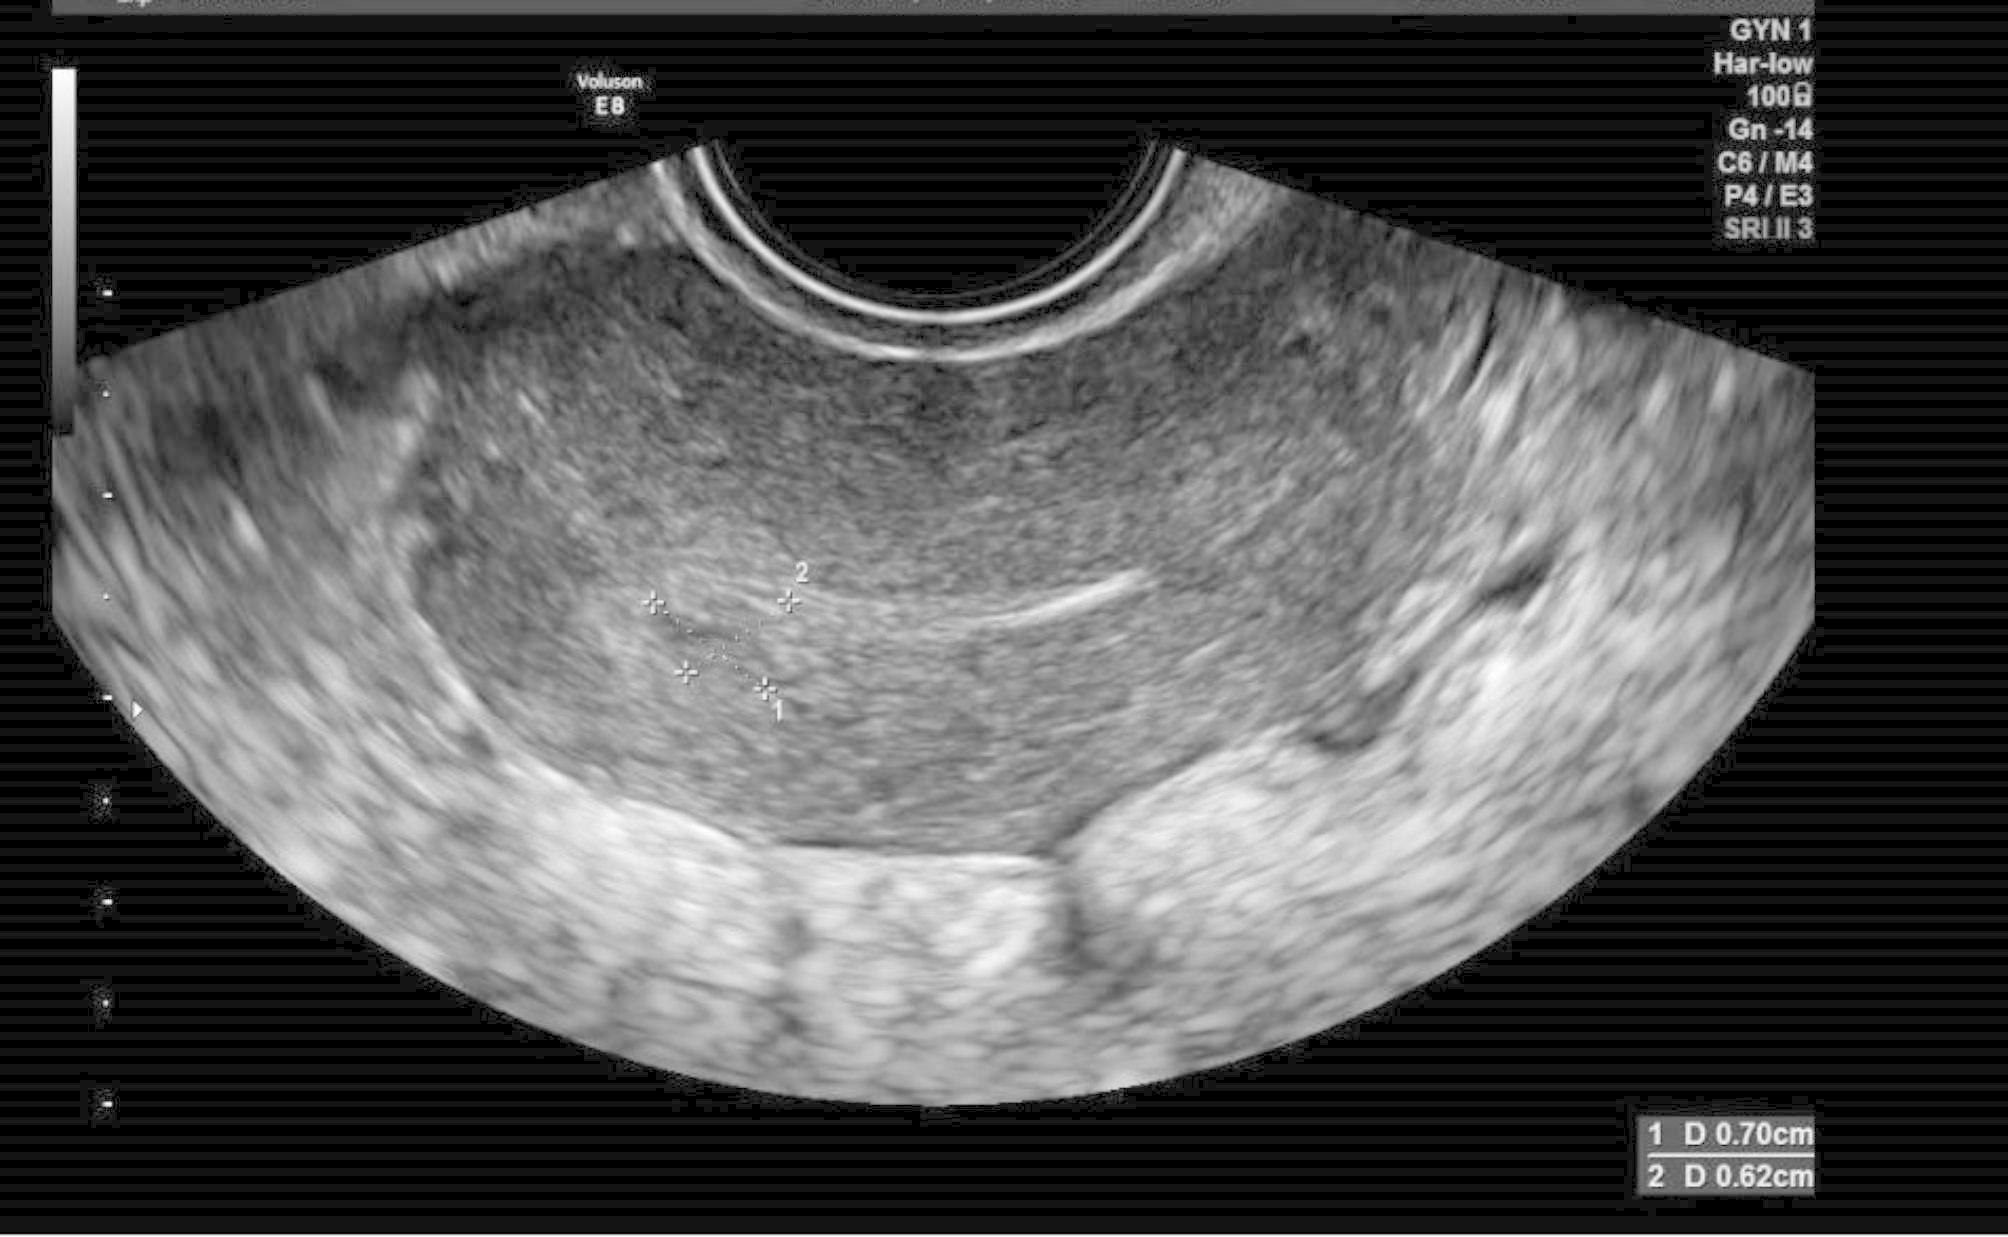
Sonographic scan for RPOC with positive pathology result. An ultrasound scan which reveals a 7 mm postpartum RPOC in an asymptomatic patient, with a positive pathological result confirming the presence of intrauterine residual remnant
